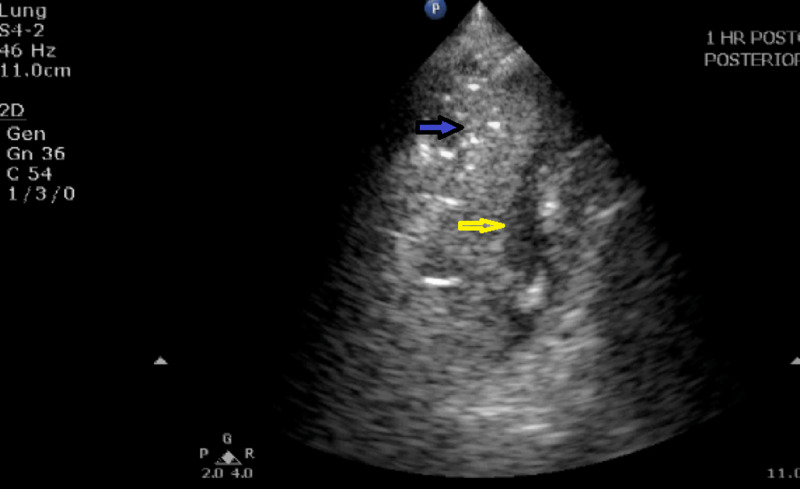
Lung ultrasound image showing consolidation and pleural effusion at T1 The figure shows consolidation (blue arrow) and a small pleural effusion (yellow arrow) at T1 (one hour post-surgery).
